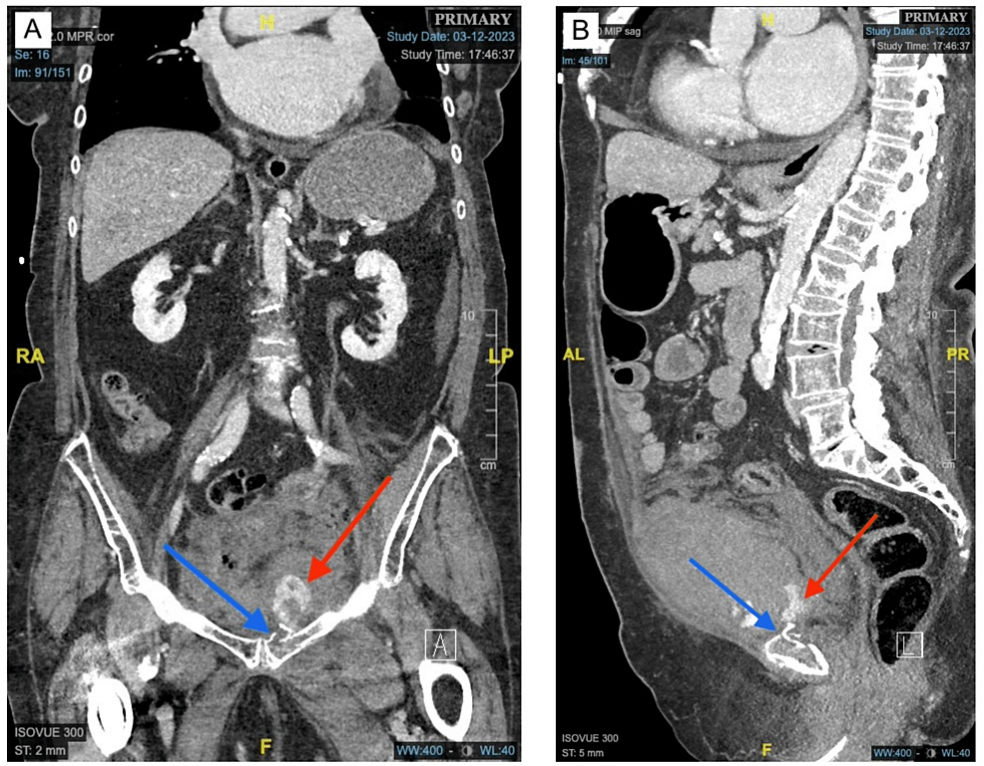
CT angiography showing active pelvic bleeding. (A) Coronal view of the left pubic body and superior pubic ramus fracture (blue arrow) and resultant active internal bleeding (red arrow). (B) Sagittal view of left pubic body fracture (blue arrow) with resultant active internal bleeding (red arrow). The nondisplaced fractures of the right inferior pubic ramus and right sacral wing are not shown in these images.
